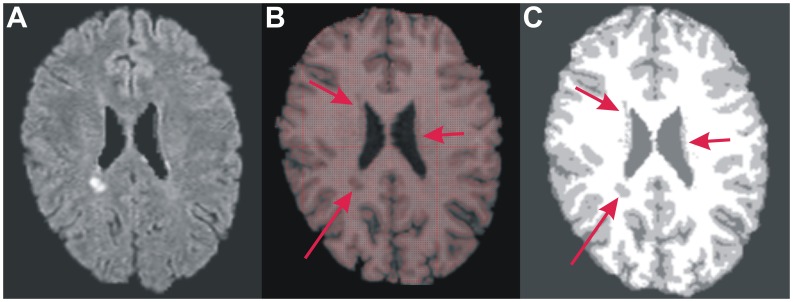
An example of automated volumetric assessment. A, FLAIR image from a patient with the usual extent of T2 hyperintense lesions seen in patients with clinically isolated syndrome. B, T1-weighted image with hypointense T1 lesions (arrows, lesion volume = 0.3 cm^3^). It is apparent that the T1 lesions were included in calculation of the overall brain volume (red area). C, T1-seighted image segmented by SIENAX. The T1 hypointense lesions (arrows) were misclassified into grey matter.
